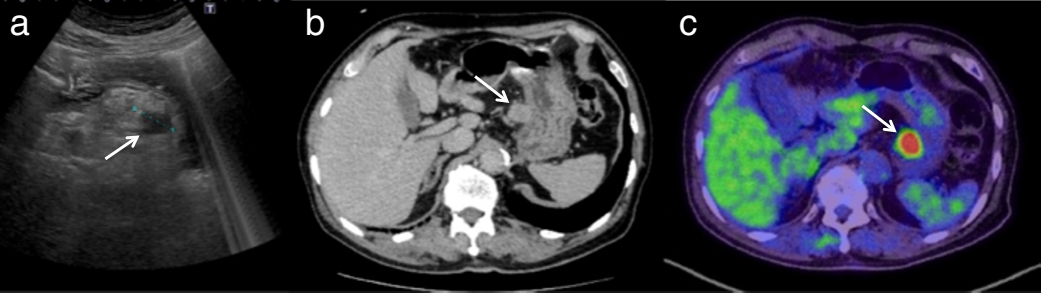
Abdominal ultrasonography (AUS), computed tomography (CT) and positron emission tomography-computed tomography (PET-CT) findings of the tumor. (a) Abdominal ultrasonography showed the tumor 20 mm in size along the lesser curvature of the stomach. (b) CT scan showed the tumor 20 mm in size at the same lesion. (c) PET-CT showed an FDG hot uptake at the same lesion.
